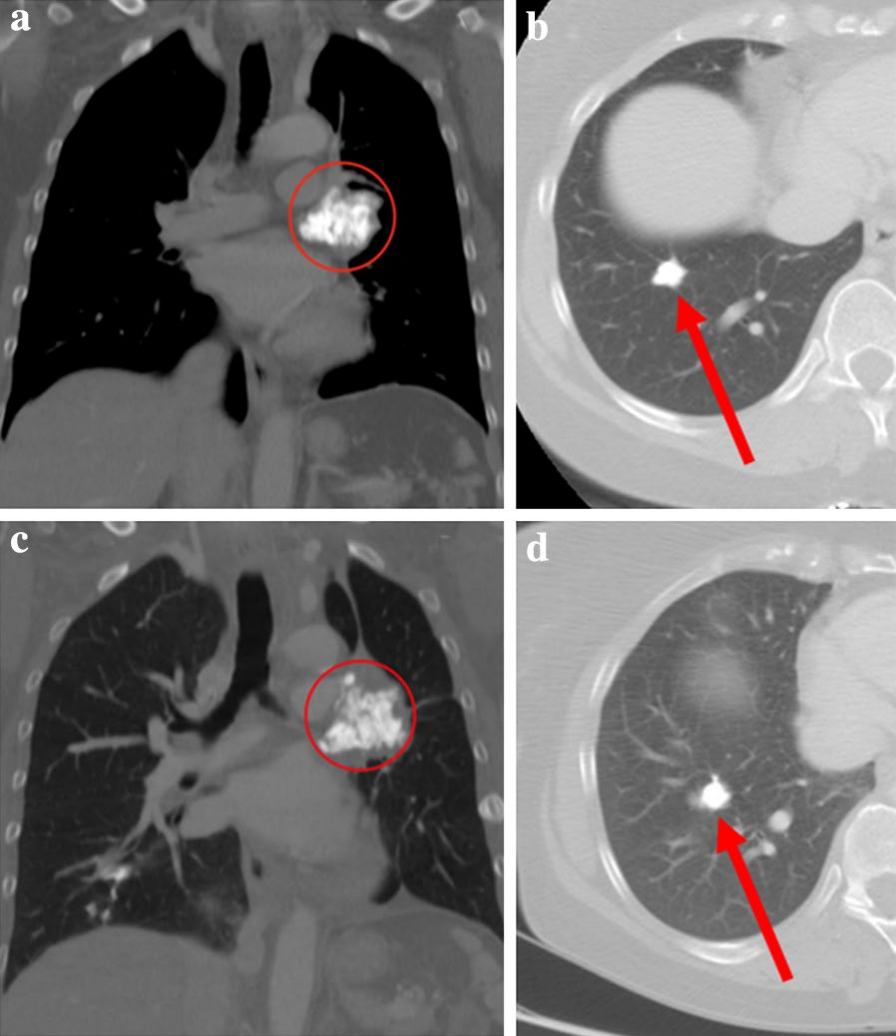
a Coronal CT image on 3/31/11 showing a calcified *left hilar*
